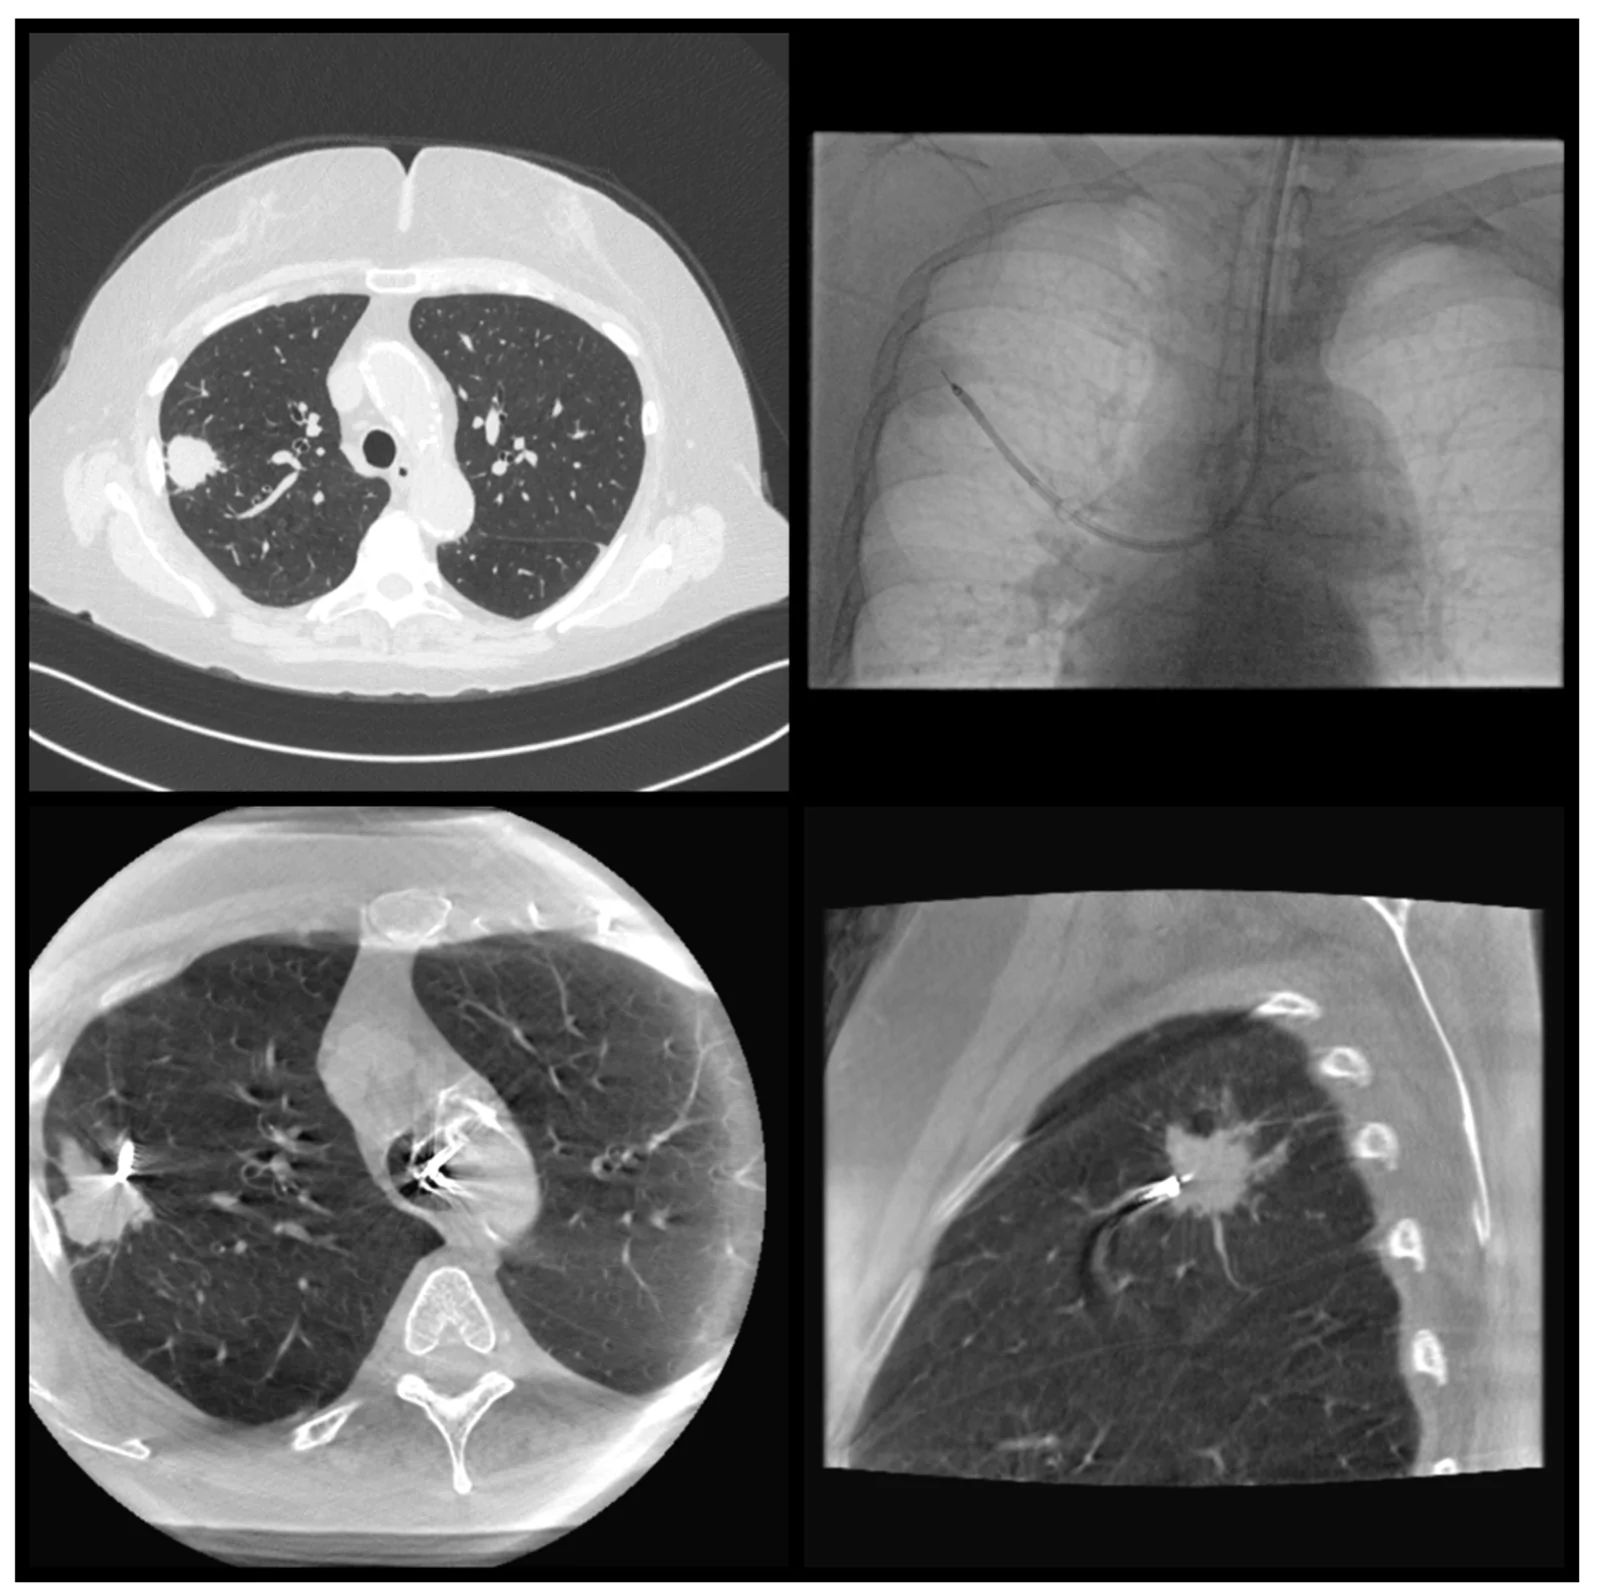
Example of the use of cone-beam CT to confirm the catheter position with “tool-in-lesion” for this right upper lobe nodule.
